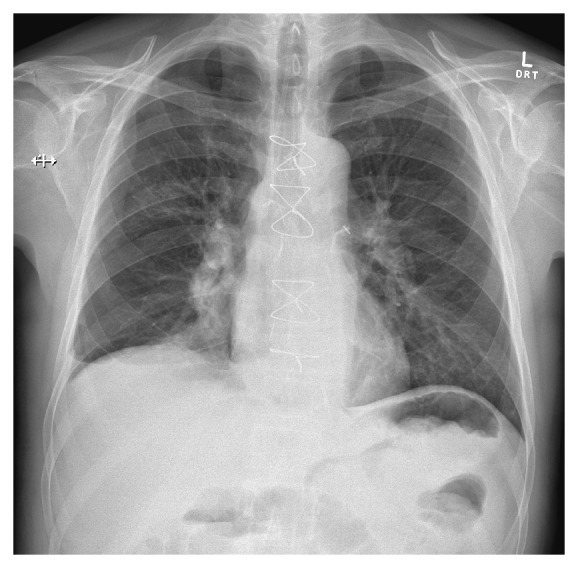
Postbronchoscopy chest X-ray.
